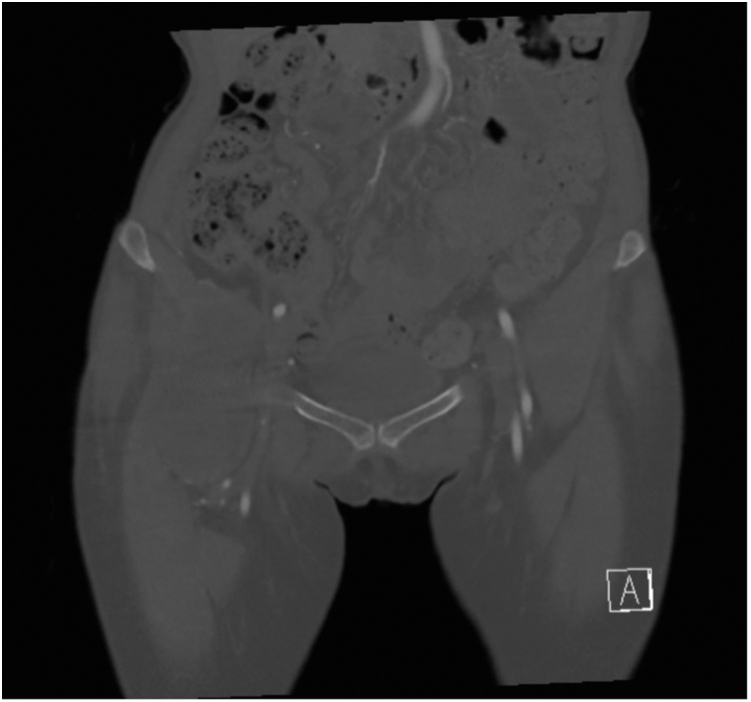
Coronal view of right-sided iliopsoas hematoma. CT scan demonstrating a hypodense mass measuring up to 5.4 × 4.9 cm in cross-section and 9.7 cm in craniocaudal dimension.
